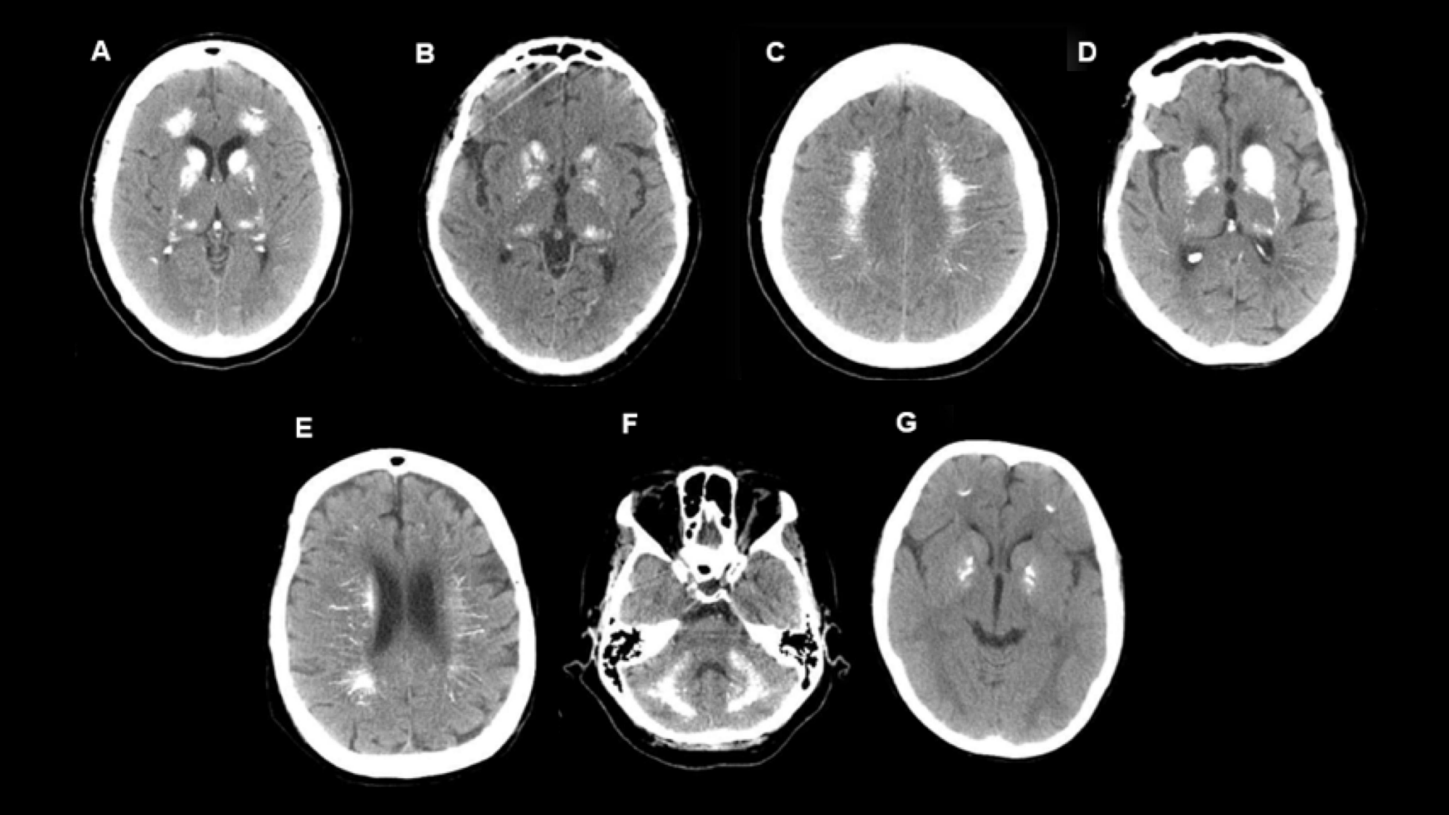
Cranial CT - Case 1 (A, B, and C): sparse white matter calcifications, base nucleus, thalamus, dentate nucleus, and cortical/subcortical regions in the occipital lobes. Case 2 (D and E): multiple symmetrical areas of calcifications in the supra/infratentorial deep gray matter and white matter in the perivascular and central pons territories. Case 3 (F): diffuse calcifications in the supratentorial white matter, base nucleus, thalamus, dentate nucleus, and some cortical/subcortical regions in the occipital lobes. Case 4 (G): calcifications in lentiform nucleus, heads of caudate nucleus, subcortical nucleus in frontal lobes, and cerebellar hemispheres.
